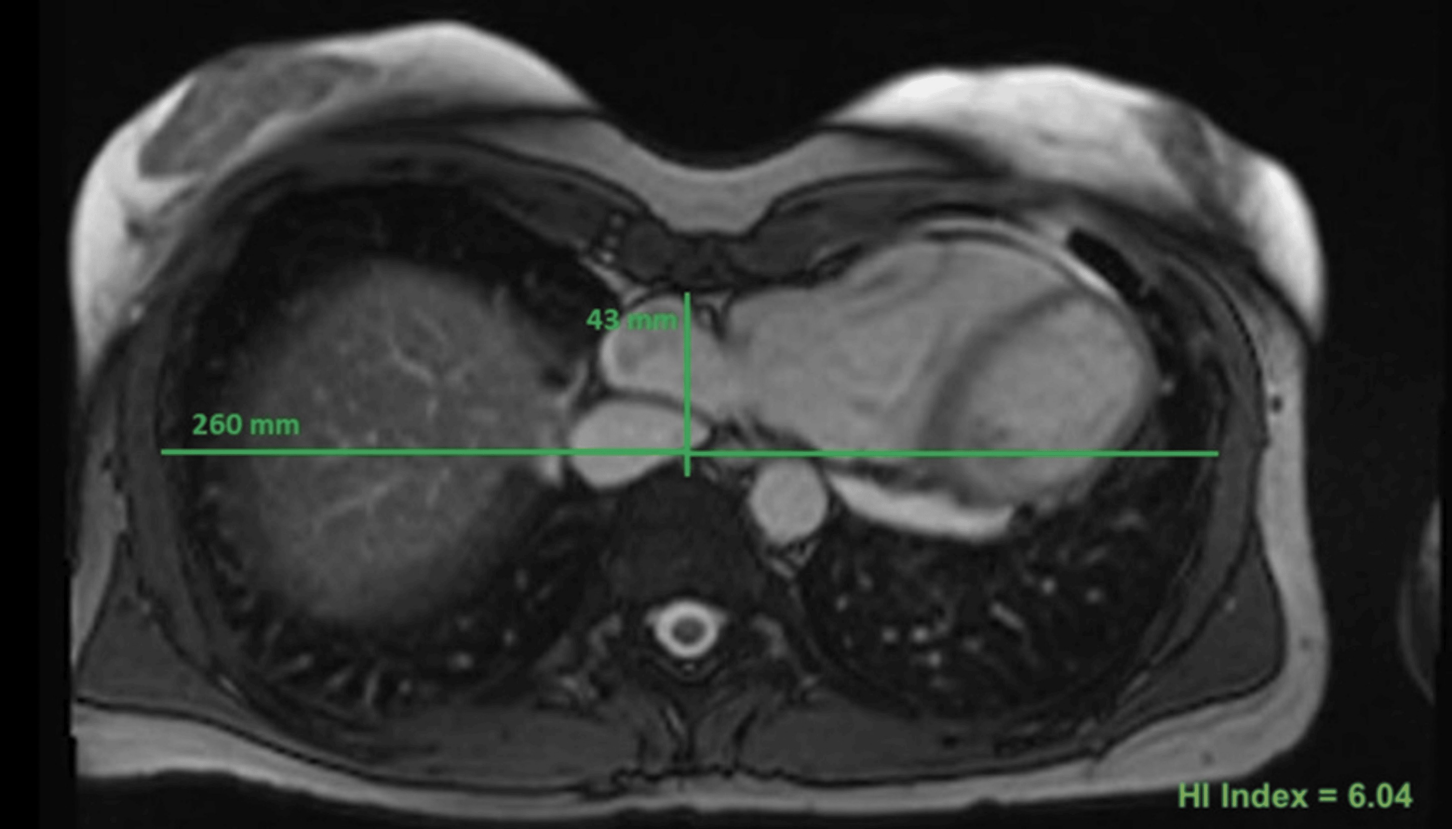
Cardiac magnetic resonance imaging depicting cardiac displacement and compression of right-sided cardiac chambers and a Haller index of 6.
